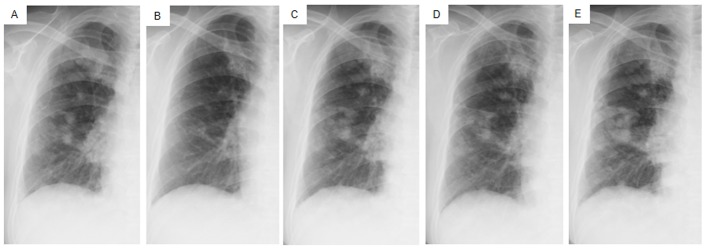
Chest X-ray films of the right lung field taken late in March 2011 before the administration of gefitinib (A), taken early in May at 1.5 months after initiating gefitinib and just before the suspension of gefitinib due to grade 3 elevation of alanine aminotransferase levels (B), taken early in June at the resumption of gefitinib on alternative days (C), taken in mid-July at the 1.5 months after the resumption of gefitinib (D), taken in mid-August after the documentation of progressive disease and 4 days after discontinuation of gefitinib (E).
